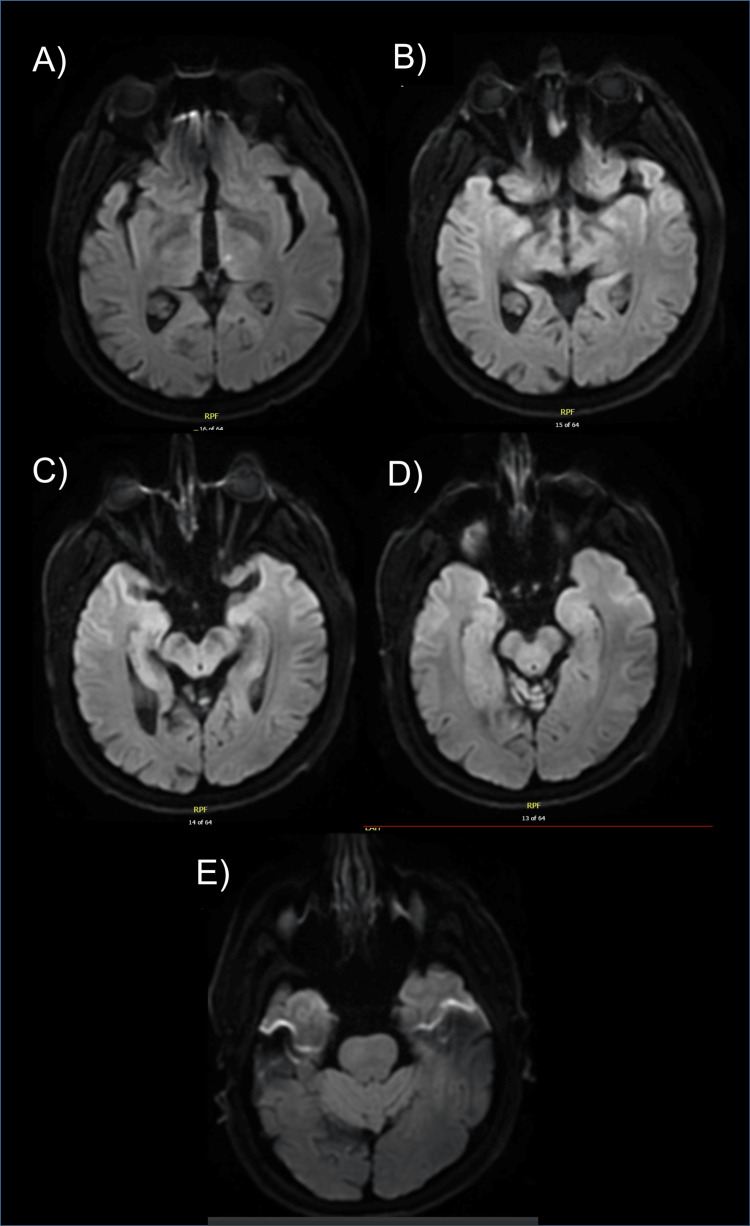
Axial DWI MRI images of midbrain and pons in order from most cranial (A) to most caudal (E). DWI: Diffusion-weighted imaging
